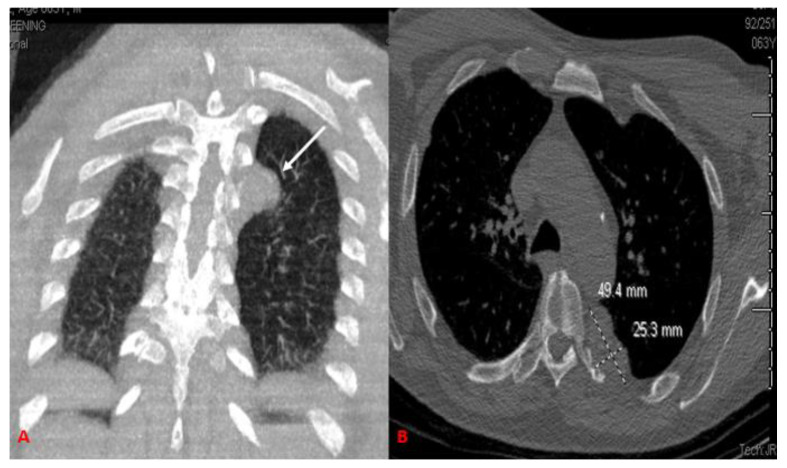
Non-contrast CT scan of the chest. Coronal (A) images show a well-circumscribed lobulated, homogeneous extrapleural mass (arrow) adjacent to the left 6th rib posteriorly. Bone window (B) shows subtle erosive changes at the left costovertebral junction adjacent to the mass. The homogeneous mass completely surround costovertebral junction.
